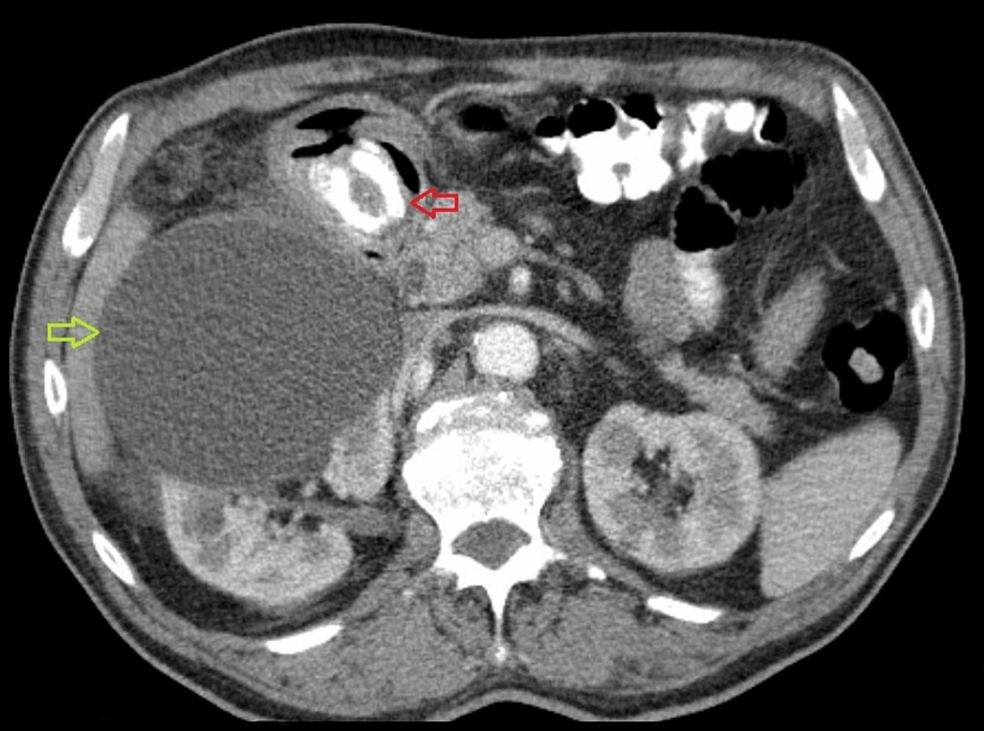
Abdominal CT (axial scan) Gallstone in the pyloric area of the stomach (red arrow) and large right kidney cyst (yellow arrow) can be seen.
